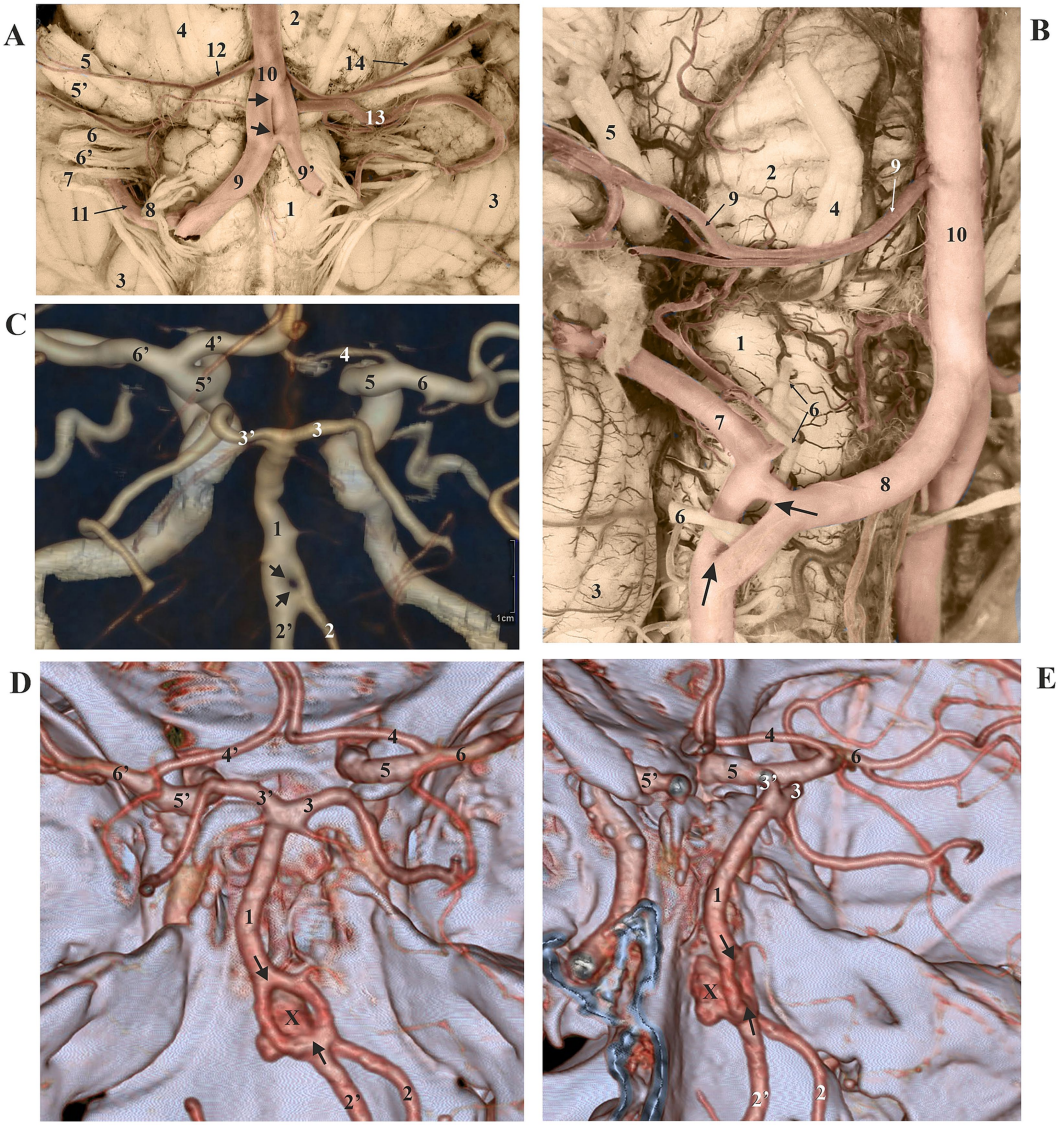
(A) Ventral view of the medulla (1), pons (2), and cerebellum (3), displaying the roots of the right abducens nerve (4), facial nerve, and vestibulocochlear nerves (5 and 5′); glossopharyngeal and vagus nerves (6 and 6′); accessory nerve (7); and hypoglossal nerve (8). The right and left vertebral arteries, VA (9 and 9′), partially merge in a slit-like fenestration (arrows) to form the BA (10). 11, right posterior inferior cerebellar artery (PICA) passing between the superior and inferior roots of the hypoglossal nerve (8); 12, right anterior inferior cerebellar artery (AICA); 13, left PICA; 14, left AICA. The arteries were injected. (B) Right ventrolateral view of the medulla (1), pons (2), and cerebellum (3), showing the roots of the right abducens nerve (4) (pierced by the pontine vein), the right facial nerve, the vestibulocochlear nerves (5), and the rootlets of the right hypoglossal nerve (6). The right PICA (7) arises from the lateral component of the fenestration (arrows) of the right VA (8), which contains the inferior roots of the hypoglossal nerve. 9, right AICA; 10, BA. Arteries were injected. (C) Dorsal view of the BA (1), featuring a fenestration (between two arrows). 2 and 2′, right (hypoplastic) and left VA; 3 and 3′, right and left P1 segments of the PCA; 4 and 4′, right (hypoplastic) and left A1 segments of the ACA; 5 and 5′, right and left ICA; 6 and 6′, right and left M1 segments of the MCA. CT angiogram. (D) Dorsal view of the BA (1), showing a significant fenestration (between two arrows) and a saccular aneurysm (X) originating from its proximal segment. 2 and 2′, right and left VA; 3 and 3′, right and left P1 segments of the PCA; 4 and 4′, right and left A1 segments of the ACA; 5 and 5′, right and left ICA; 6 and 6′, right and left M1 segments of the MCA. CT angiogram. (E) Left lateral view of the BA (1), displaying a large fenestration (between two arrows) and a saccular aneurysm (X) arising from its proximal segment, extending anteriorly and upwards. 2 and 2′, right and left VA; 3 and 3′, right and left P1 segments of the PCA; 4, right A1 segments of the ACA; 5 and 5′, right and left ICA; 6, right M1 segments of the MCA. CT angiogram.
